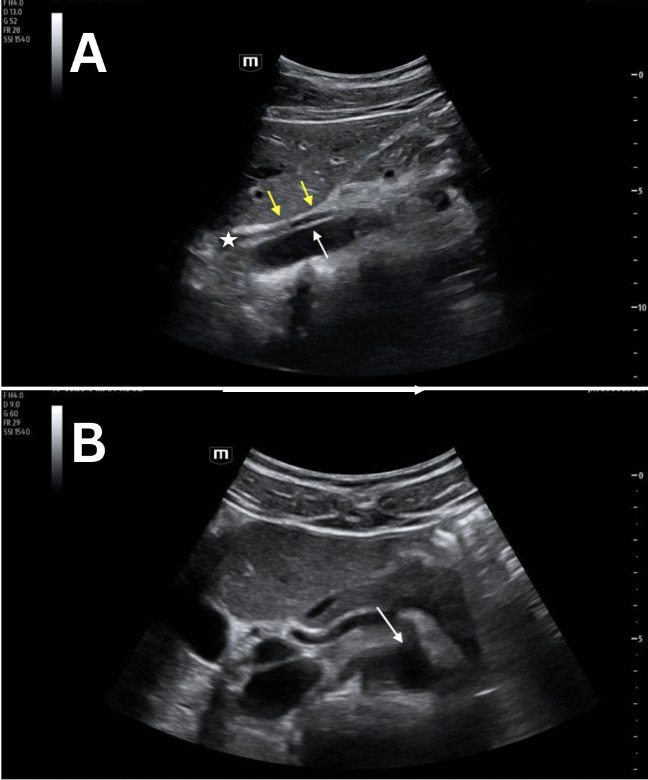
Grayscale point of care ultrasound (POCUS) images of a 22-year-old female standardized patient showing (A) sagittal view with two neighboring linear, hyperechoic structures (double yellow arrow and white arrow) and the intervening, hypoechoic crus of the diaphragm (white star) and (B) transverse view with a normal, anechoic lumen of the abdominal aorta (white arrow).
